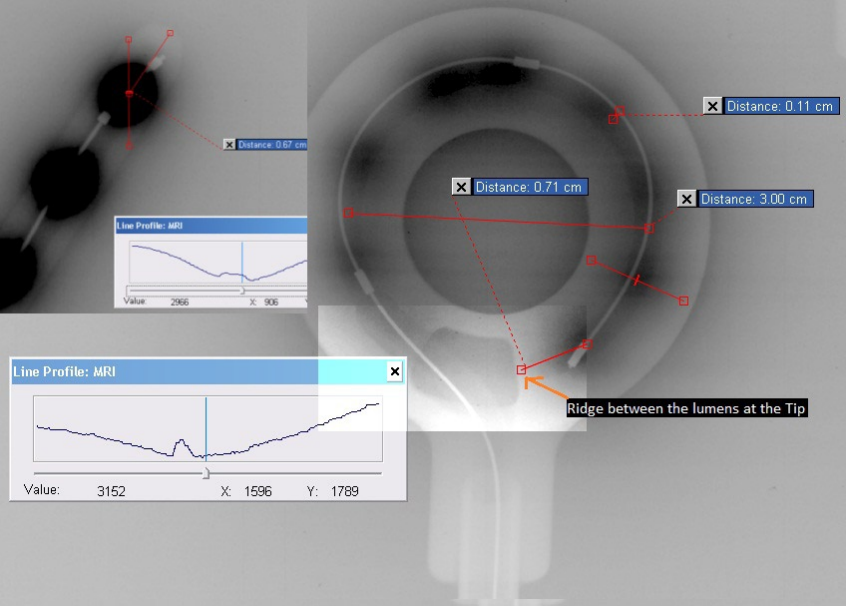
Autoradiographs of Nucletron ring applicators set for source positions identification. Figure 1(a) shows the distances of the first dwell position from the middle point of the ridge between the air channel trends (lumen of the applicator) at the tip of ring applicator as 0.71 cm. The deviation of radio‐opaque dummy source from the center of dwell position of radiation source shows as 0.11 cm. Inset figure shows the definition of first dwell position from the middle point of the ridge between the inner air channel and outer surface of applicator at the tip using the Full width at half of the maximum CT number of line profile drawn on a line passing through the center (dwell position) of the source.
